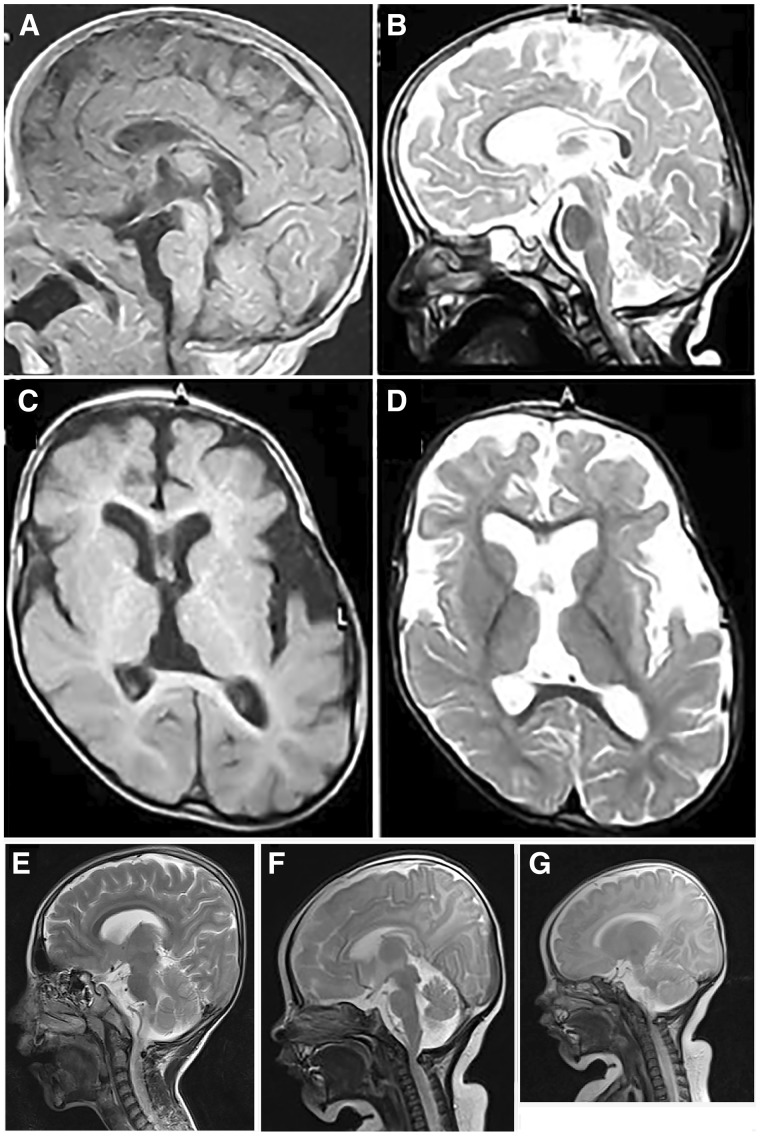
Brain MRI. (A–D) Case 3 (Patient B-IV:4). Sagittal T_1_ and T_2_ cuts through the midline at the age of 1 month (A) and 2 years (B) showing hypoplastic corpus callosum, mainly body and to a lesser degree its genu, progressive cerebellar and cerebral atrophy and a cervical notch. Axial cuts T_1_ (C) and T_2_ (D) at the age of 2 years demonstrating mild-to-moderate cortical atrophy predominating on left hemisphere and mildly enlarged lateral ventricles. (E–G) Cases 7 and 8 (Patients D-V:2 and D-V:3). (E) Sagittal MRI image of Case 7 (Patient D-V:2) showing prominent inner liquor spaces without overt brain malformations. (F) Sagittal MRI image of Case 8 (Patient D-V:3) showing prominent inner liquor spaces without apparent brain malformations except a cervical notch causing an impression in the upper cervical spinal cord area. (G) Normal cerebellar size is demonstrated in Case 8 (Patient D-V: 3).
